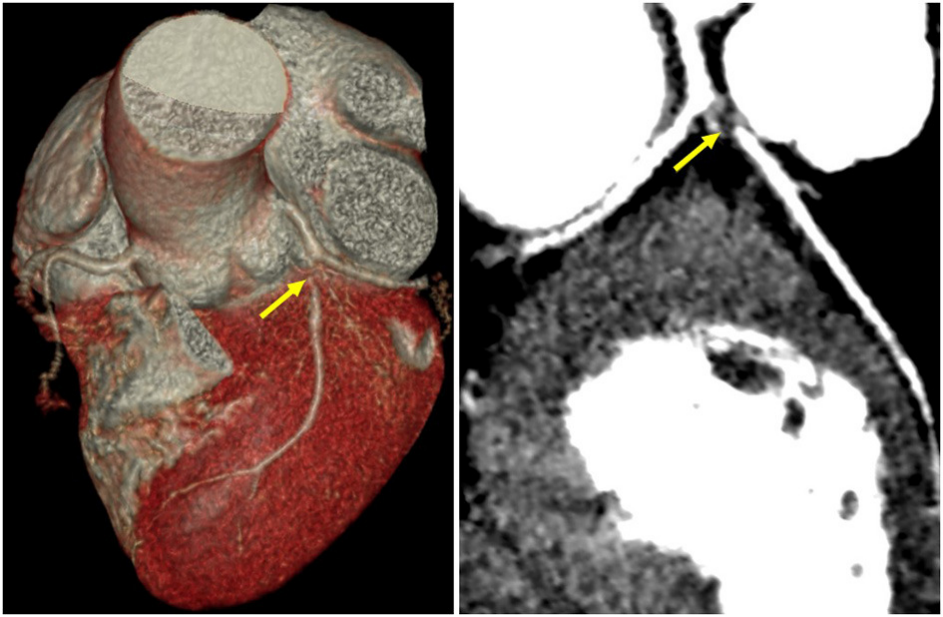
Coronary CT angiography revealed critical stenosis of the left anterior descending artery (yellow arrows).
